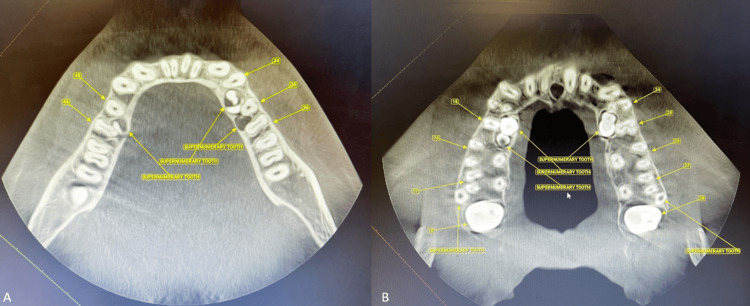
CBCT scan showing impacted teeth in respective arches. (A) Axial view - mandible, (B) Axial view - maxilla CBCT- Cone beam CT
